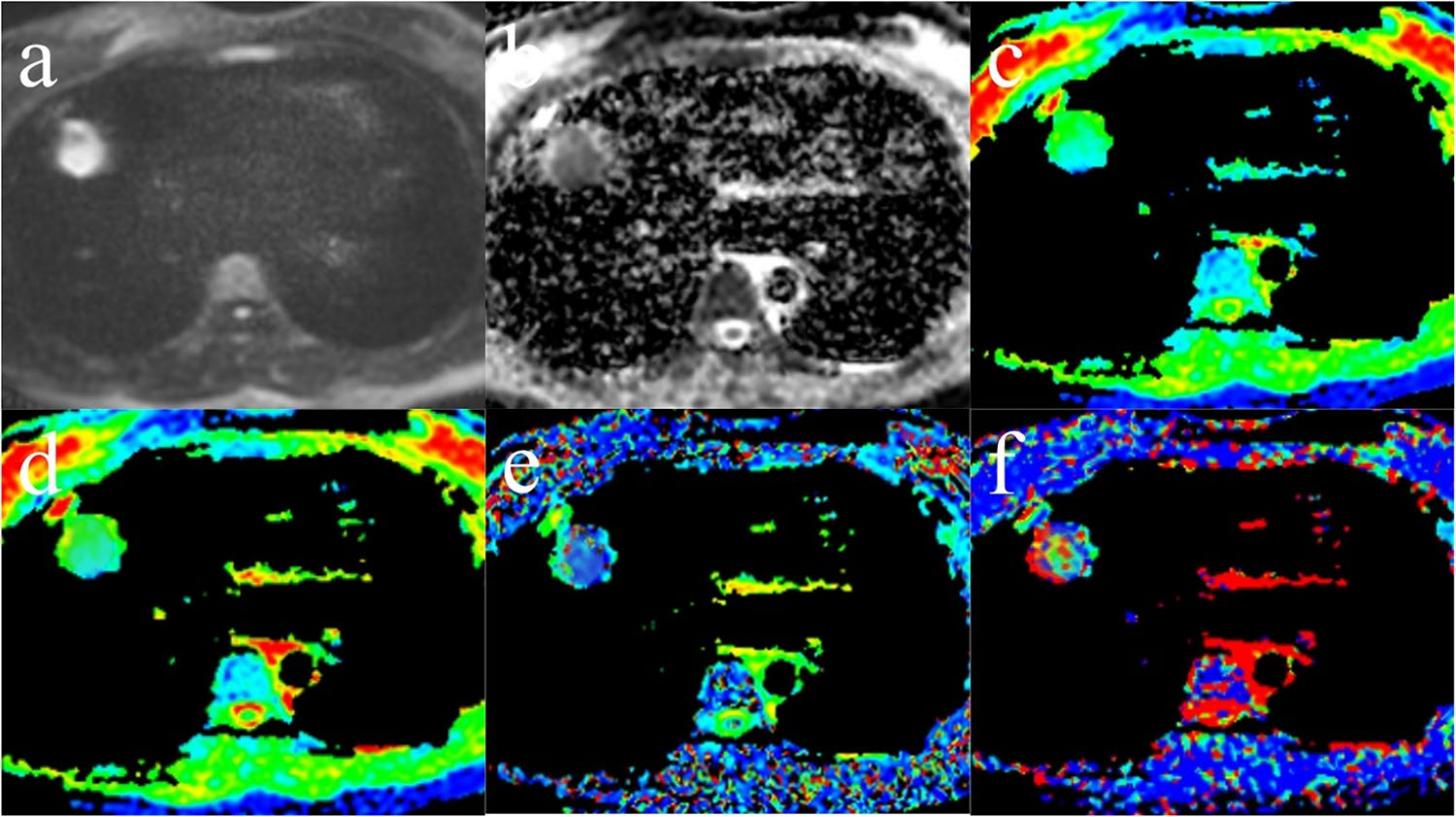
Pulmonary invasive adenocarcinoma in the right middle lobe. (a) Diffusion-weighted imaging (DWI) (*b* = 800 s/mm^2^); the signal intensity (SI) of the lesion was increased, and the lesion-spinal signal intensity ratio (LSR) was 1.230. (b) ADC_800_ map. (c) ADC_total_ map. (d) Pure diffusion coefficient (D) map. (e) Perfusion fraction (f) map. (f) Perfusion-related diffusion coefficient (D_star_) map.
